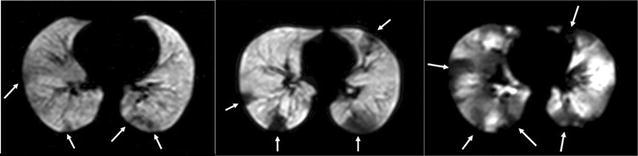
Hyperpolarized helium-3 MRI axial slice of a mild asthmatic (*left panel*), a moderate asthmatic (*middle panel*), and a severe asthmatic (*right panel*). As depicted by the *arrows*, increasing severity of disease is associated with increasing ventilation defects and ventilation heterogeneity (reproduced with permission from [28])
